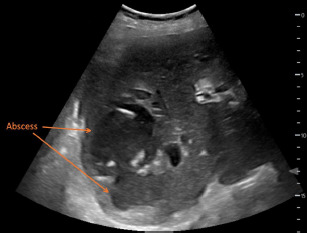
Soft tissue point-of-care ultrasound showing multiple, hypoechoic, loculated fluid collections within the parenchyma of the right lobe of the liver, indicative of hepatic abscesses.
